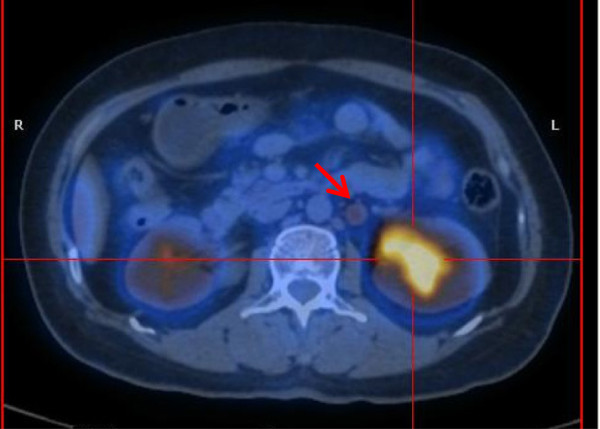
Positron emission tomography/computed tomography. This scan shows a hypermetabolic renal pelvic mass and an enlarged retroperitoneal lymph node (arrow).
